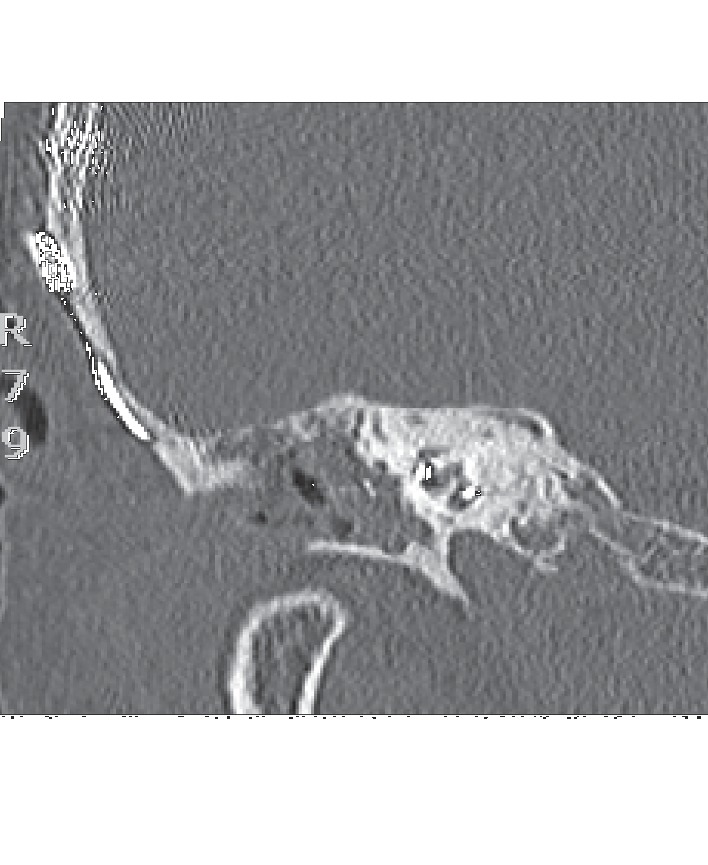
CT after revision surgery.
